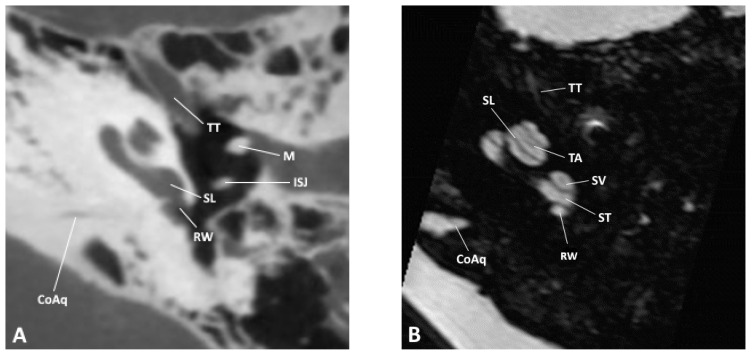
Axial slice through the inner and middle ear at the level of the round window as observed by (A) CBCT and (B) MRI; CoAq, cochlear aqueduct; ISJ, incudostapedial joint; M, malleus; RW, round window; SL, spiral lamina; ST, scala tympani; SV, scala vestibuli; TA, truncation artifact; TT, tensor tympani.
